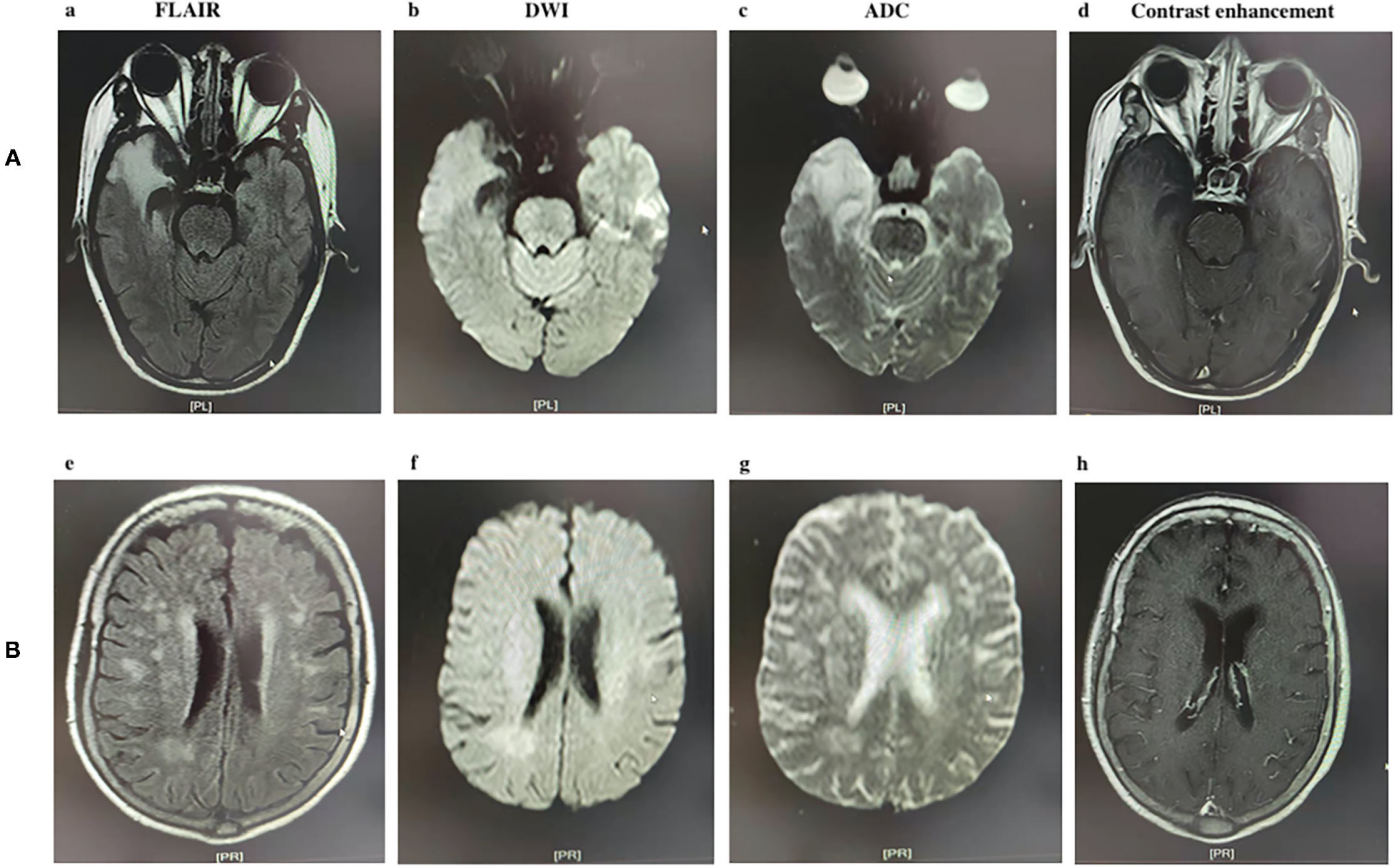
Two types of MRI image appearance in patients with anti-N-methyl-D-aspartate (NMDA) receptor encephalitis. (A) A 45-year-old male patient with lesions located in the right temporal lobe; (a) axial fluid-attenuated inversion recovery (FLAIR) image showing high signal; (b) Diffusion-weighted imaging showing slightly high signal; (c) Apparent diffusion coefficient image showing high signal, (d) no contrast enhancement is evident in this axial T1 sequence. (B) A 22-year-old female patient with lesions located in the white matter; (e) axial FLAIR image showing high signal; (f) DWI image showing slightly high signal; (g) ADC image showing slightly high signal, (h) no contrast enhancement is evident in this axial T1 sequence.
